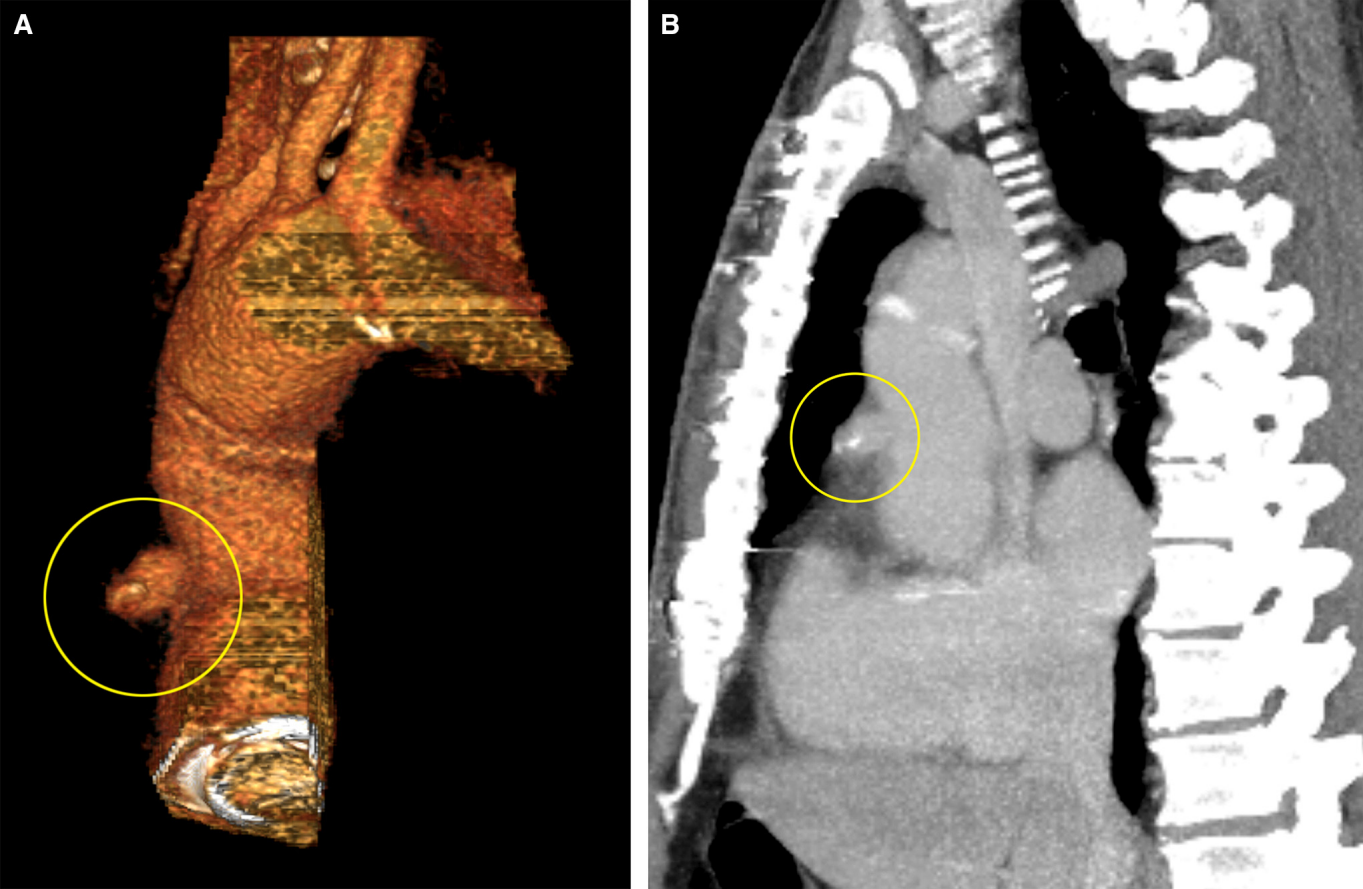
Computed tomography image showing the pseudoaneurysm (*encircled in yellow*) in a 3-dimensionally reconstructed (A) and sagittal (B) view.
